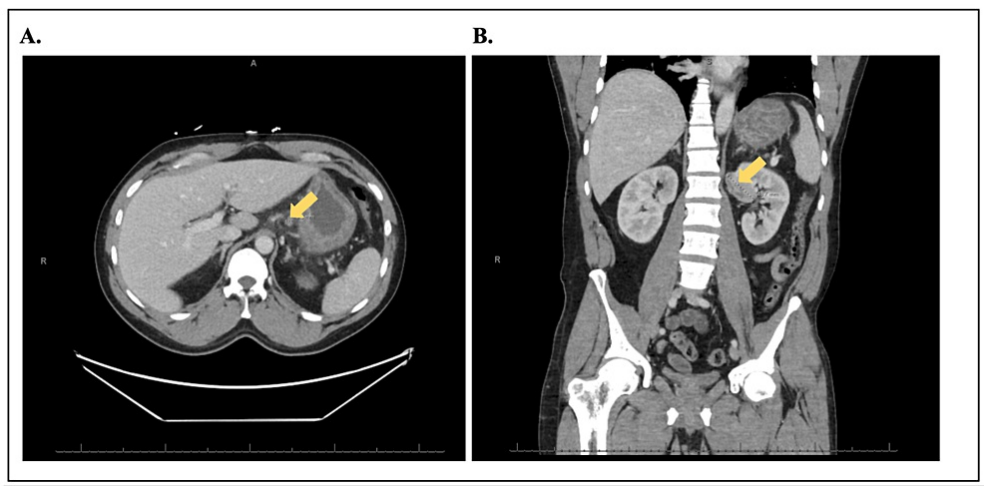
A. CT abdomen pelvis shows gastric mural edema with a diameter of 9.3 mm on day 1; B. CT abdomen pelvis shows the incidental finding of a left renal mass with a diameter of 48.7 mm on day 1
